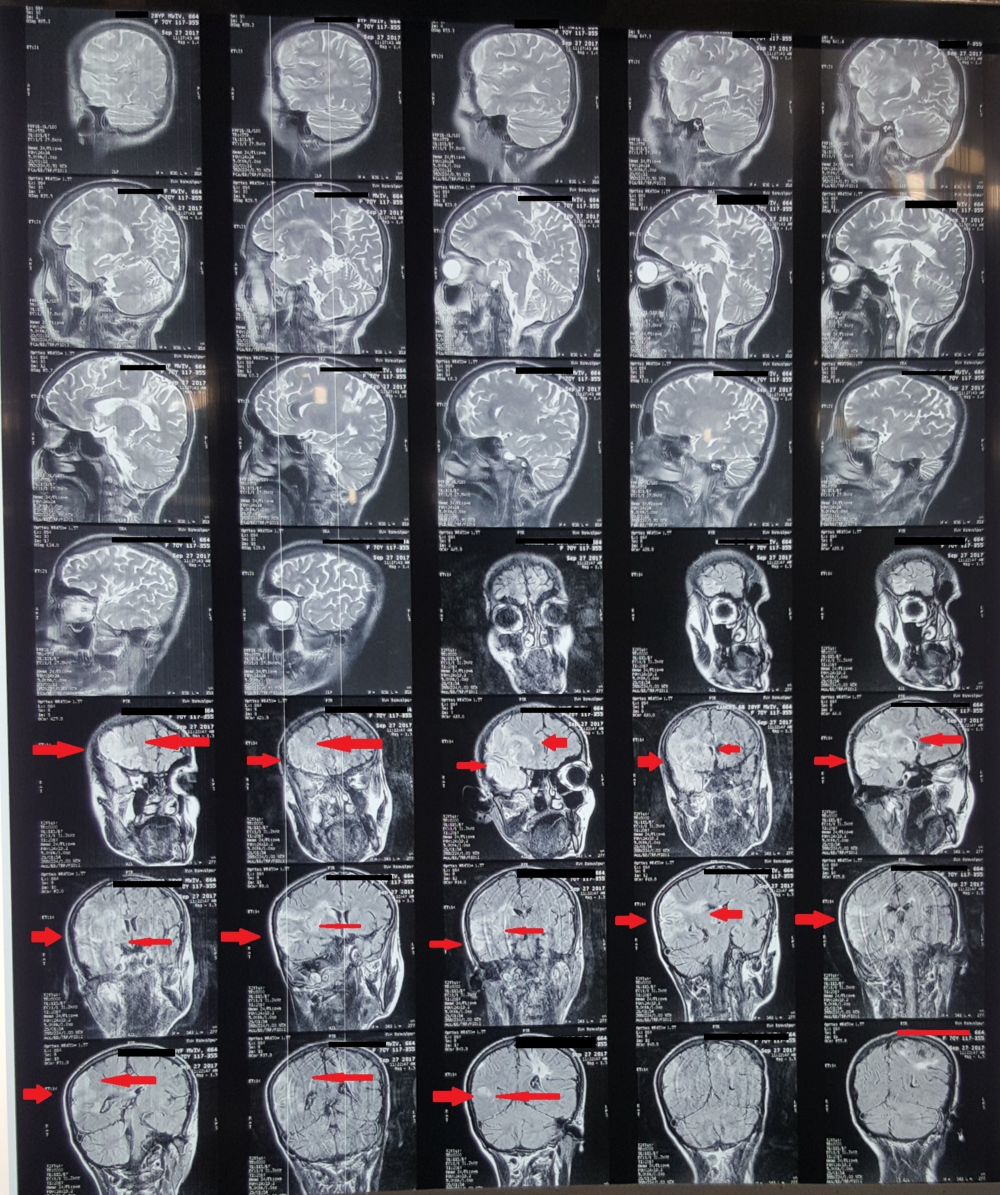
Magnetic resonance imaging (MRI) showing changes in the infarct (red arrows)
